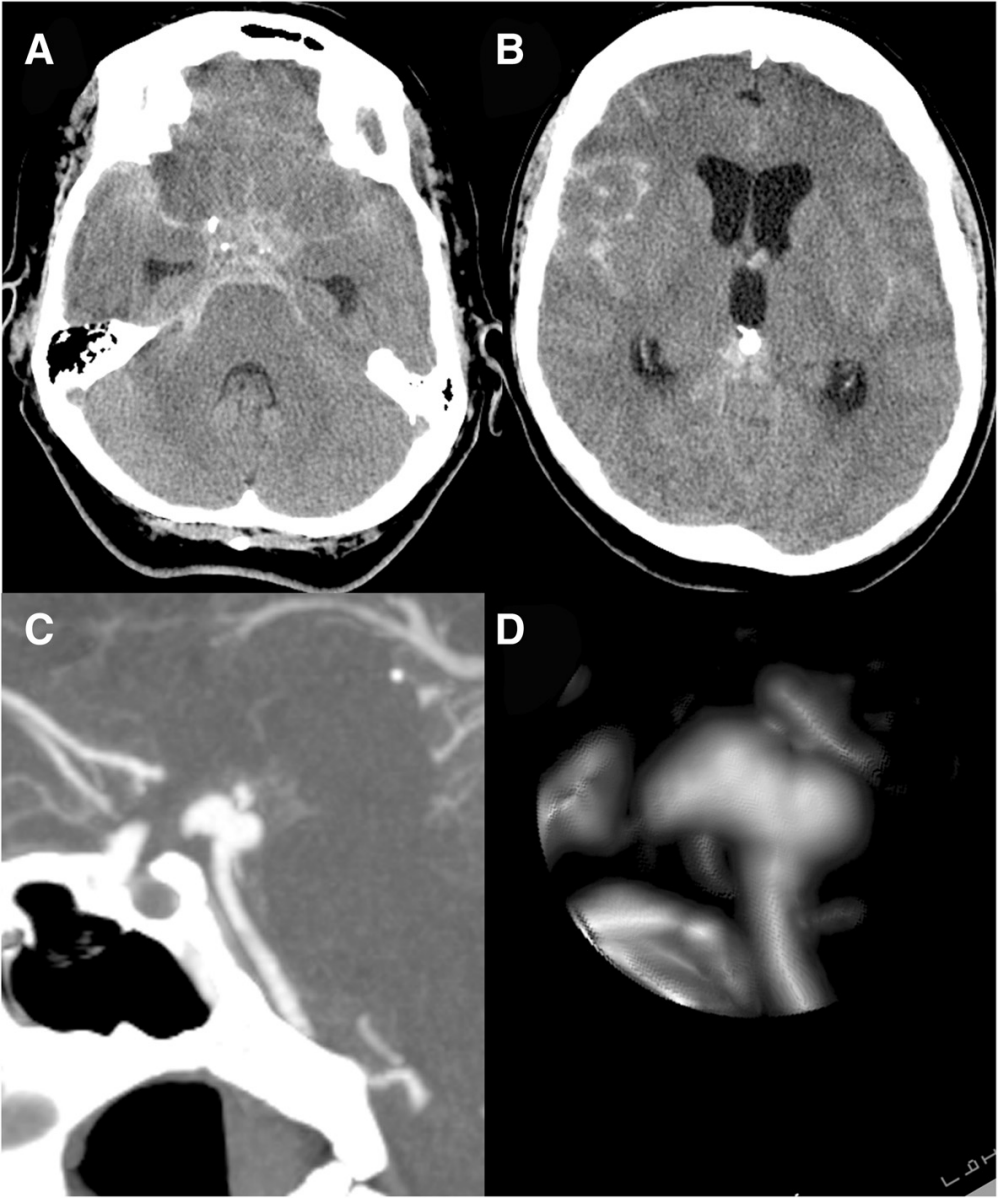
Cranial computed tomography scan 21 days after presentation. A cranial computed tomography scan 21 days after presentation showing diffuse subarachnoid hemorrhage (A) with hydrocephalus (B). The computed tomographic angiogram sagittal view (C) and lateral view of a reconstruction (D) show growth of the aneurysm on the anterior aspect.
